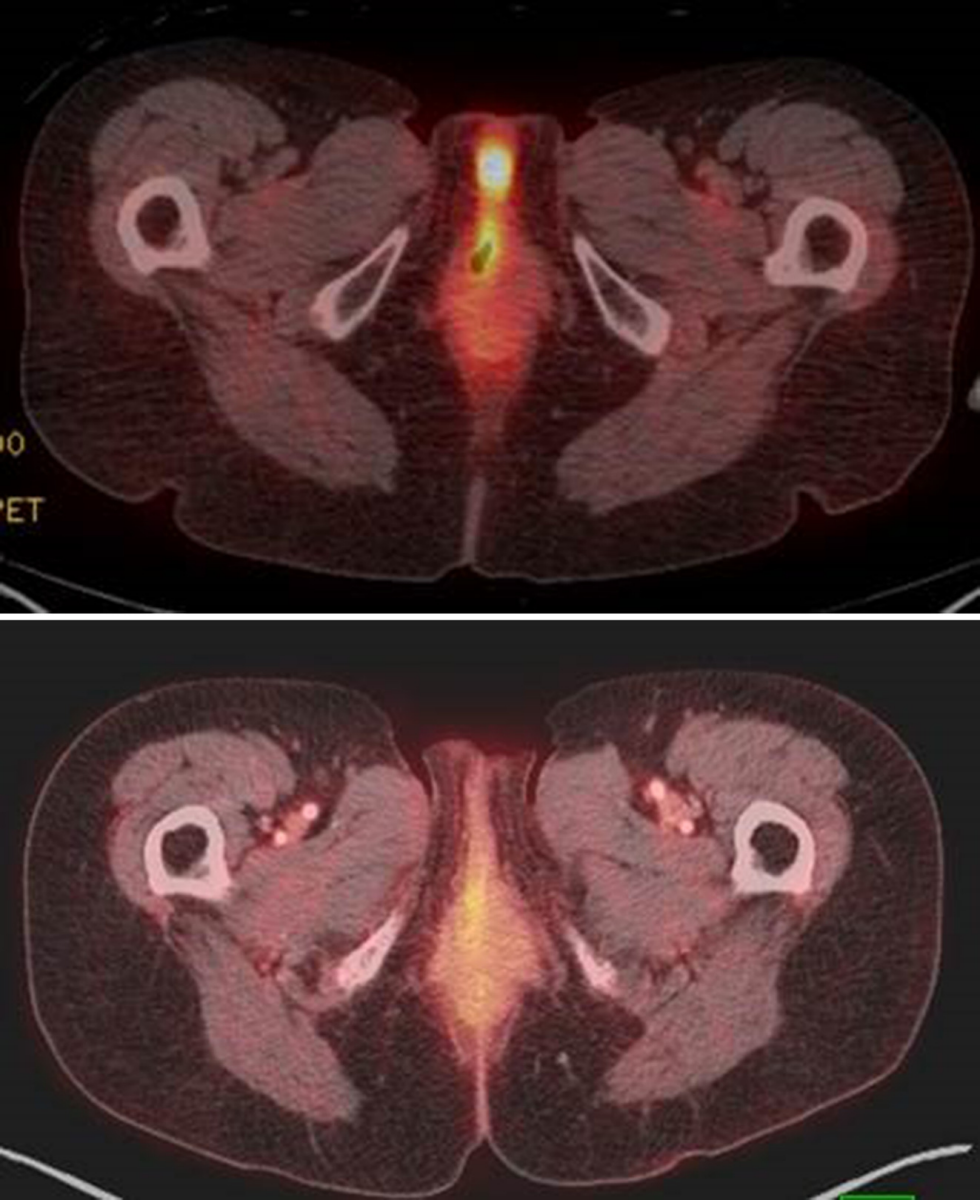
Top: Positron emission tomography/CT scan prior to the start of treatment, demonstrating a recurrent periclitoral mass. Bottom: complete resolution of the focal uptake at 15 months post treatment with radiation and ipilimumab.
